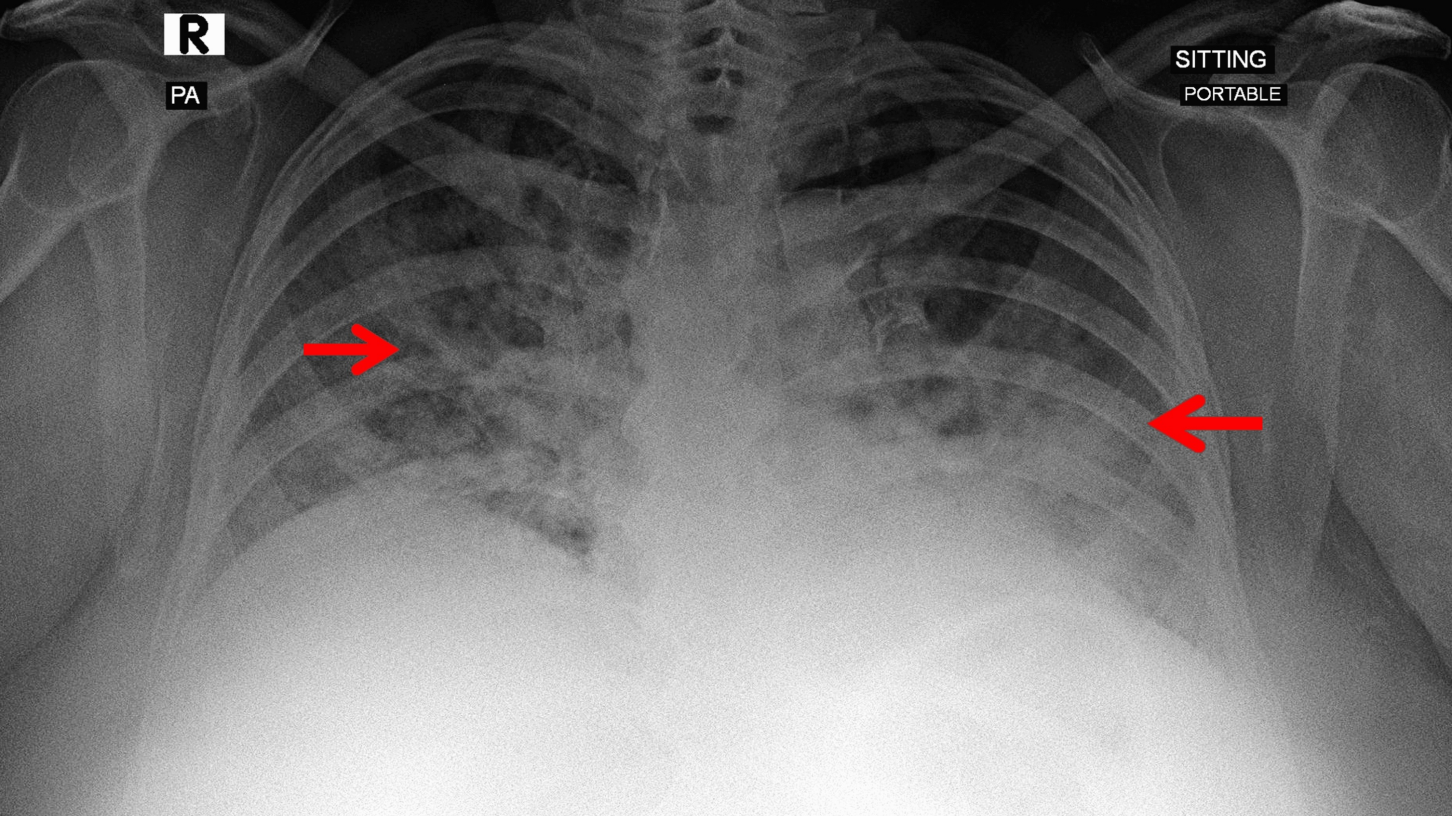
Admission chest radiograph anteroposterior (portable) view The initial chest radiograph at presentation shows patchy, bilateral airspace opacities with lower-zone predominance, consistent with acute diffuse lung involvement. Red arrows indicate representative bilateral patchy opacities.
